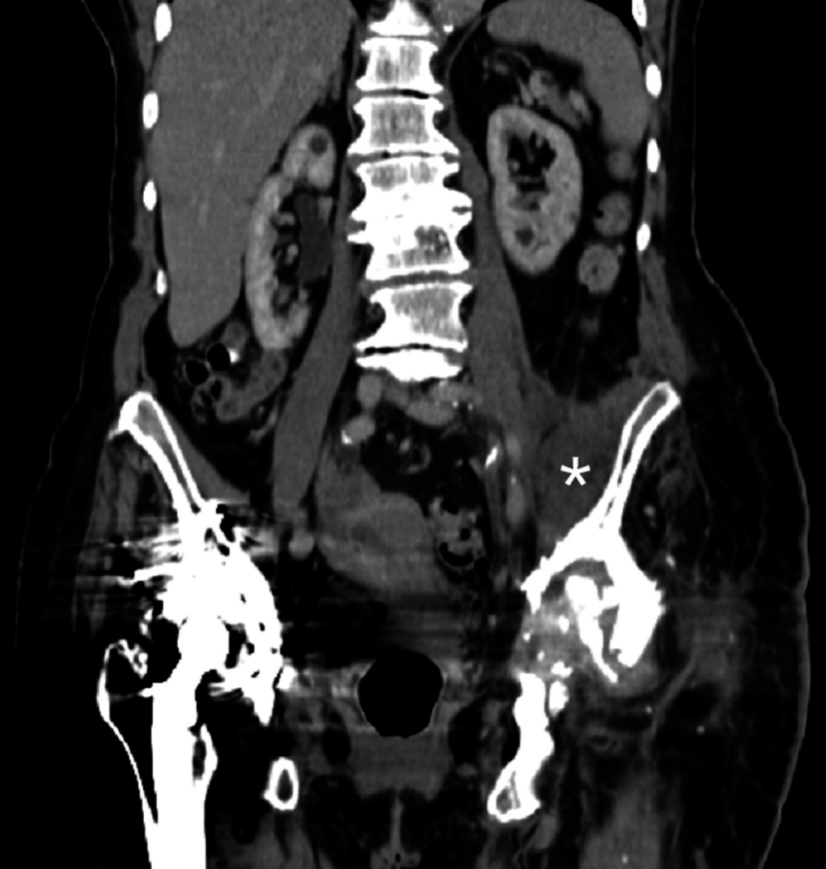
CT scan with soft tissue window showing a 7 × 5 cm left psoas abscess (asterisk). The left infected implant has been removed.
